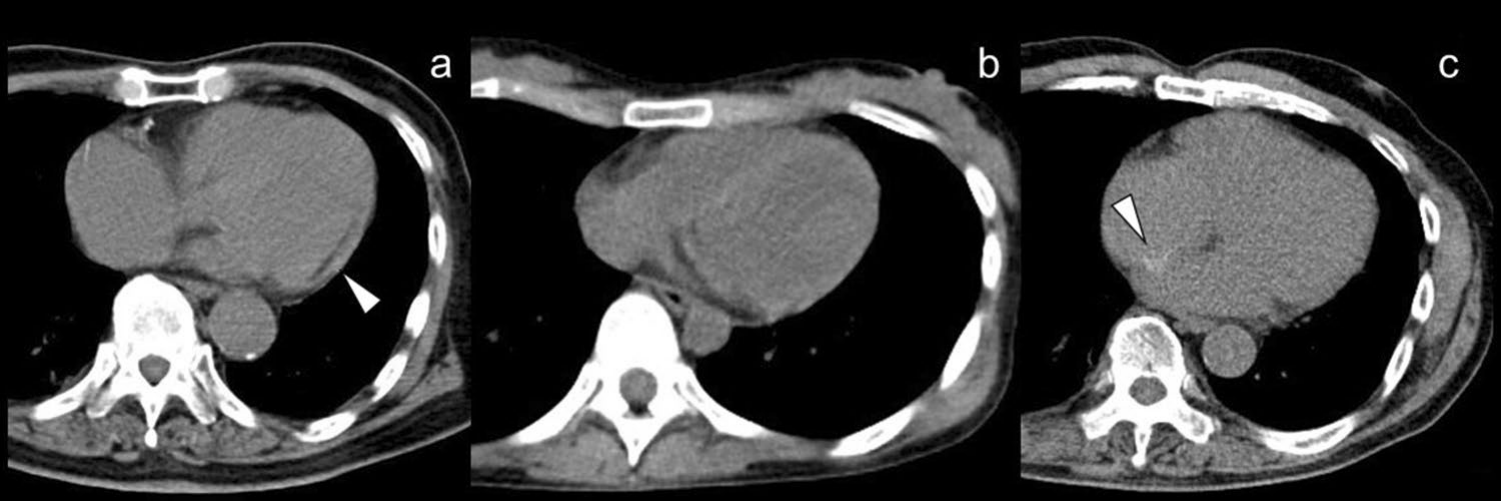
Incidental CT findings in FDG-PET/CT. A curvilinear dark line (white arrowhead) in the myocardium indicating fibrosis in patients with a history of myocardial infarction (a). The interventricular septum was visualized in patient with low hemoglobin concentration due to the decreased CT density in blood pool (b). The patient had a history of occluding interatrial septal defect (c). A high-density foci (white arrowhead) in the interatrial septal indicated the operative site
